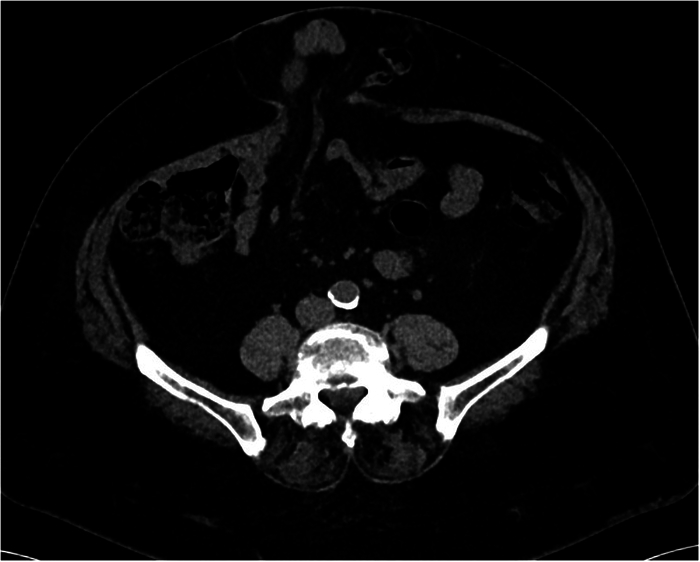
Computed tomography imaging of an umbilical hernia.
